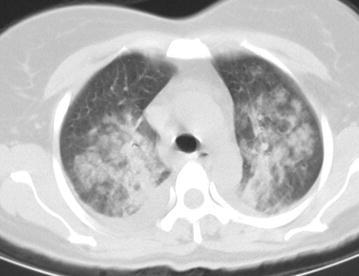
Fourty years old female patient with the alveolar densities and ground glass opacities (alveolar hemorrhage) were seen in computerized tomography (CT) scanning of the chest
